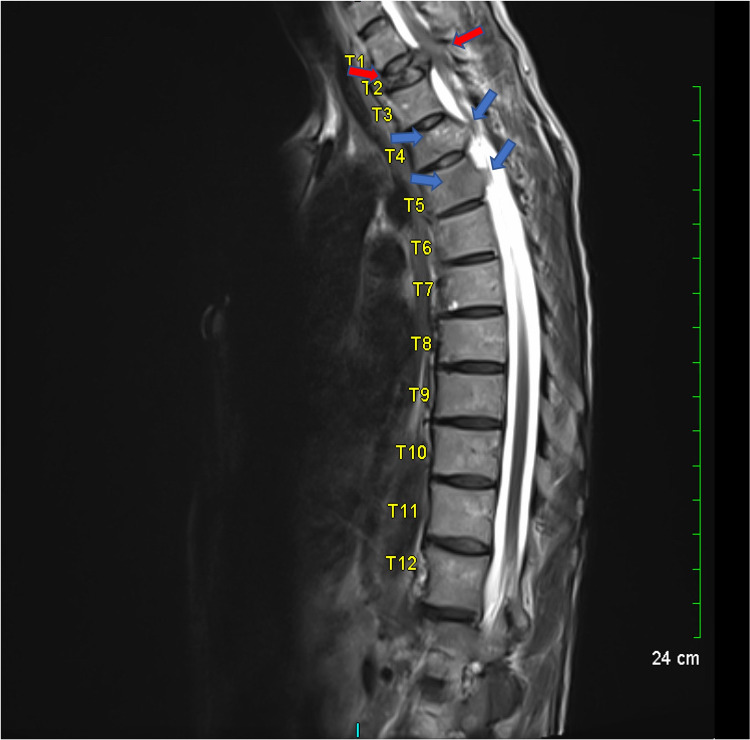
Sagittal MRI of the thoracic spine Severe compression fracture of the T2 vertebral associated with an expansile, epidural mass causing compression of the spinal cord (red arrows). An infiltrative process involving T4 and T5 vertebral bodies (blue arrows).
